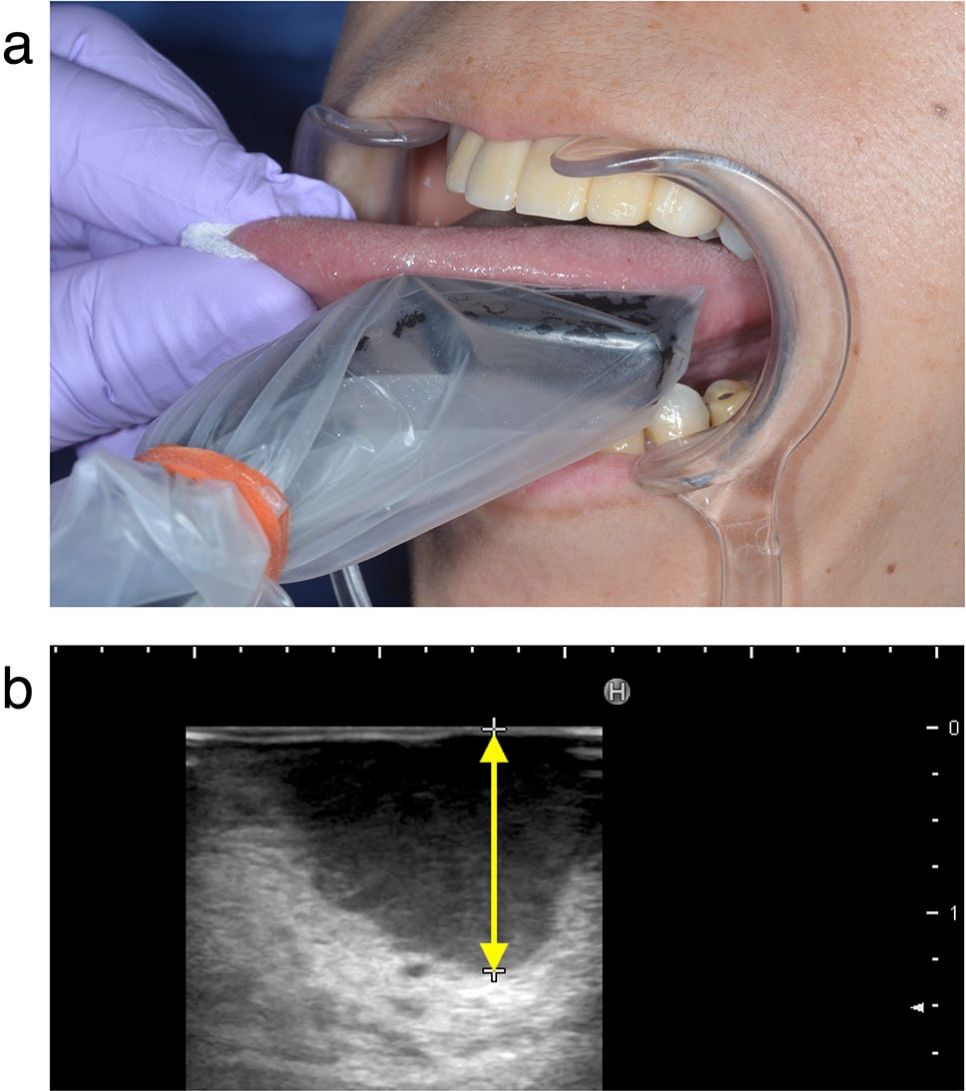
(a) Detection of the deepest portion of the tumor. Tongue was let to put forward and measured while holding its apex with gauze. (b) Measurement of r-DOI by ultrasound. The tumor was abnormally hypoechoic and the deepest point of tumor was determined. *r-DOI* radiological depth of invasion.
